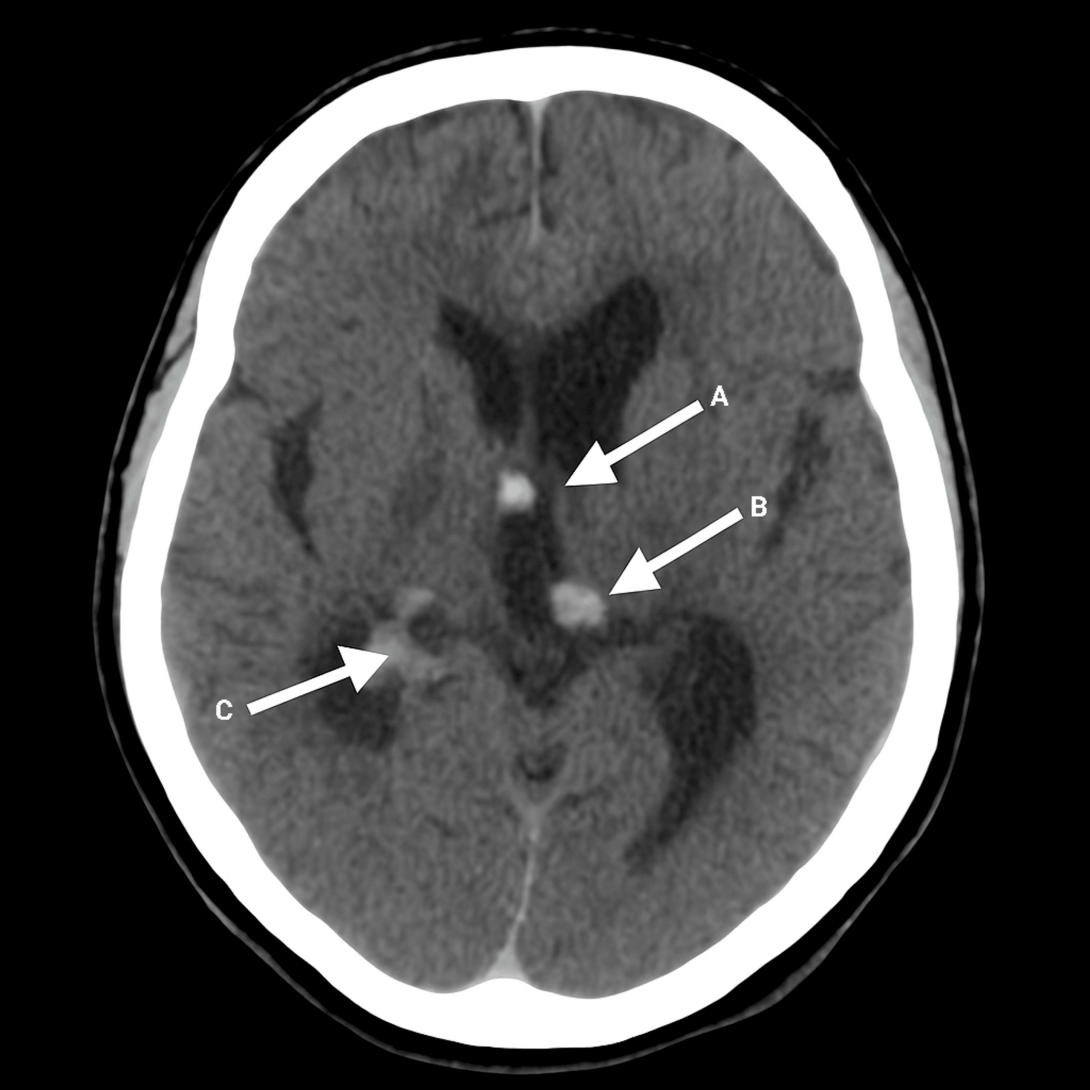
Axial Non-contrast Stealth CT Brain – Stereotactic Planning Prior to CSF Diversion. This axial CT image demonstrates a large acute intracranial hematoma centered in the left caudate nucleus (Arrow A), with intraventricular extension visible in the lateral and third ventricles (Arrow B). There is associated mild surrounding edema. Probable mild communicating hydrocephalus is suggested by prominence of the temporal horns (Arrow C). No evidence of transependymal edema or vascular malformation is noted. Moderate arterial narrowing was later confirmed on angiography, consistent with suspected vasospasm.
